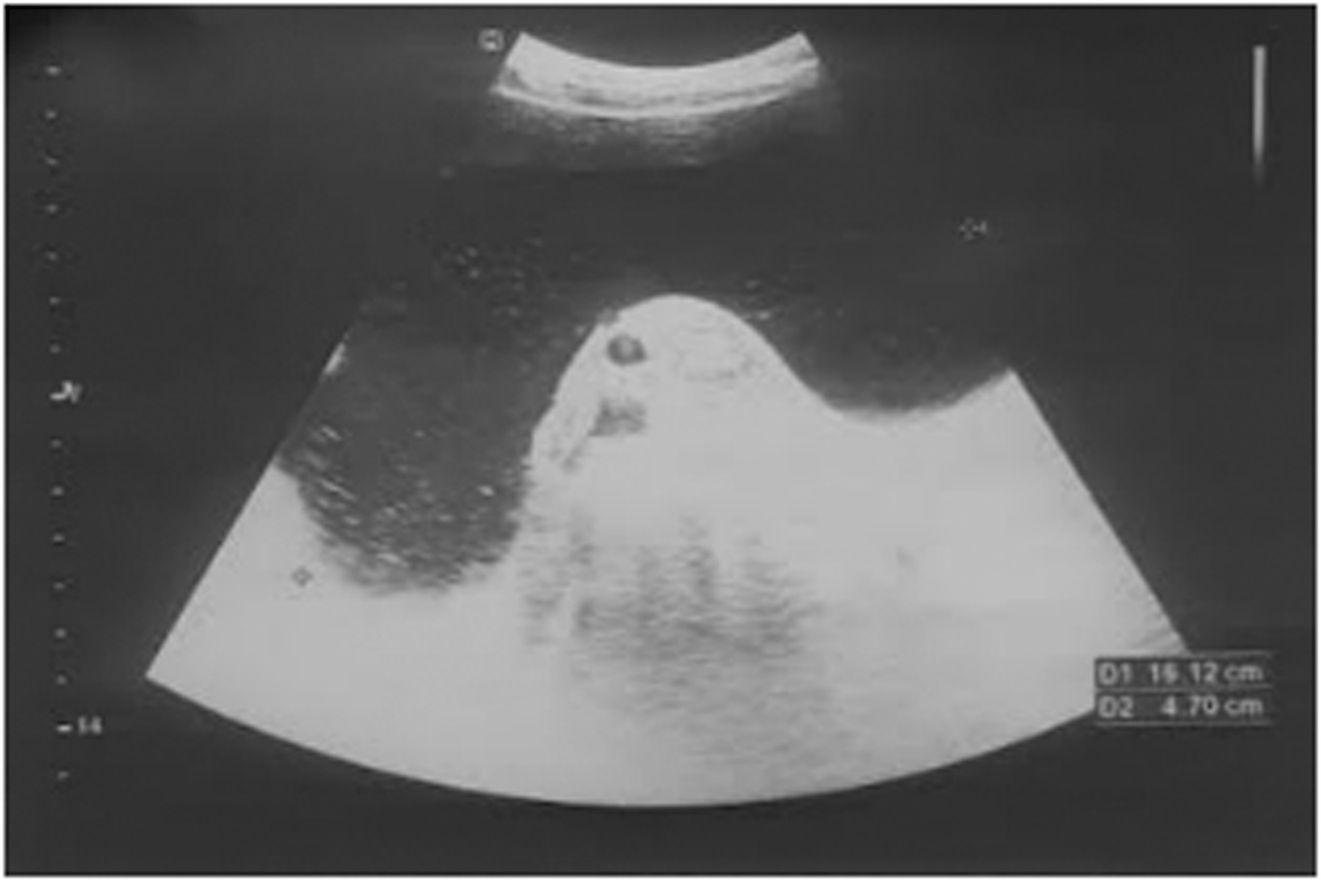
Ultrasonography of abdomen and pelvis shows cystic mass with floating echogenic debris, and no solid component and papillary projection
